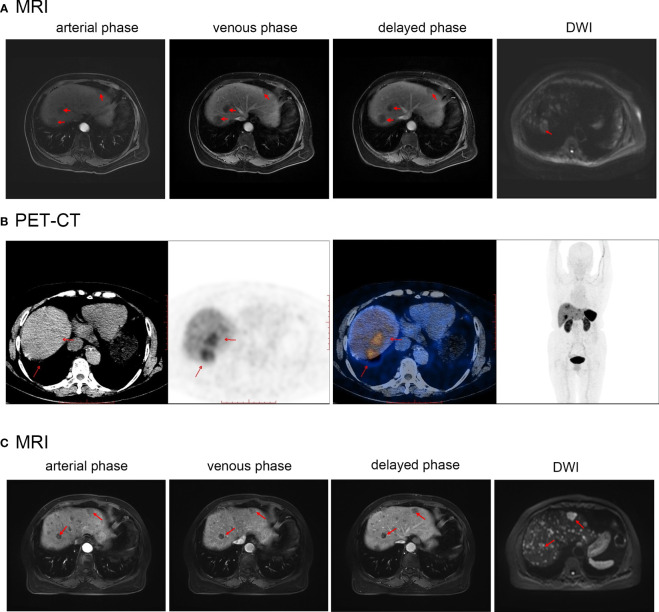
(A) Abdominal and chest CT before first treatment of Case1. (B) PET-CT before the first treatment of Case1. (C) Abdominal and chest CT after the first treatment of Case1. Red arrows indicate the location of the tumor.
